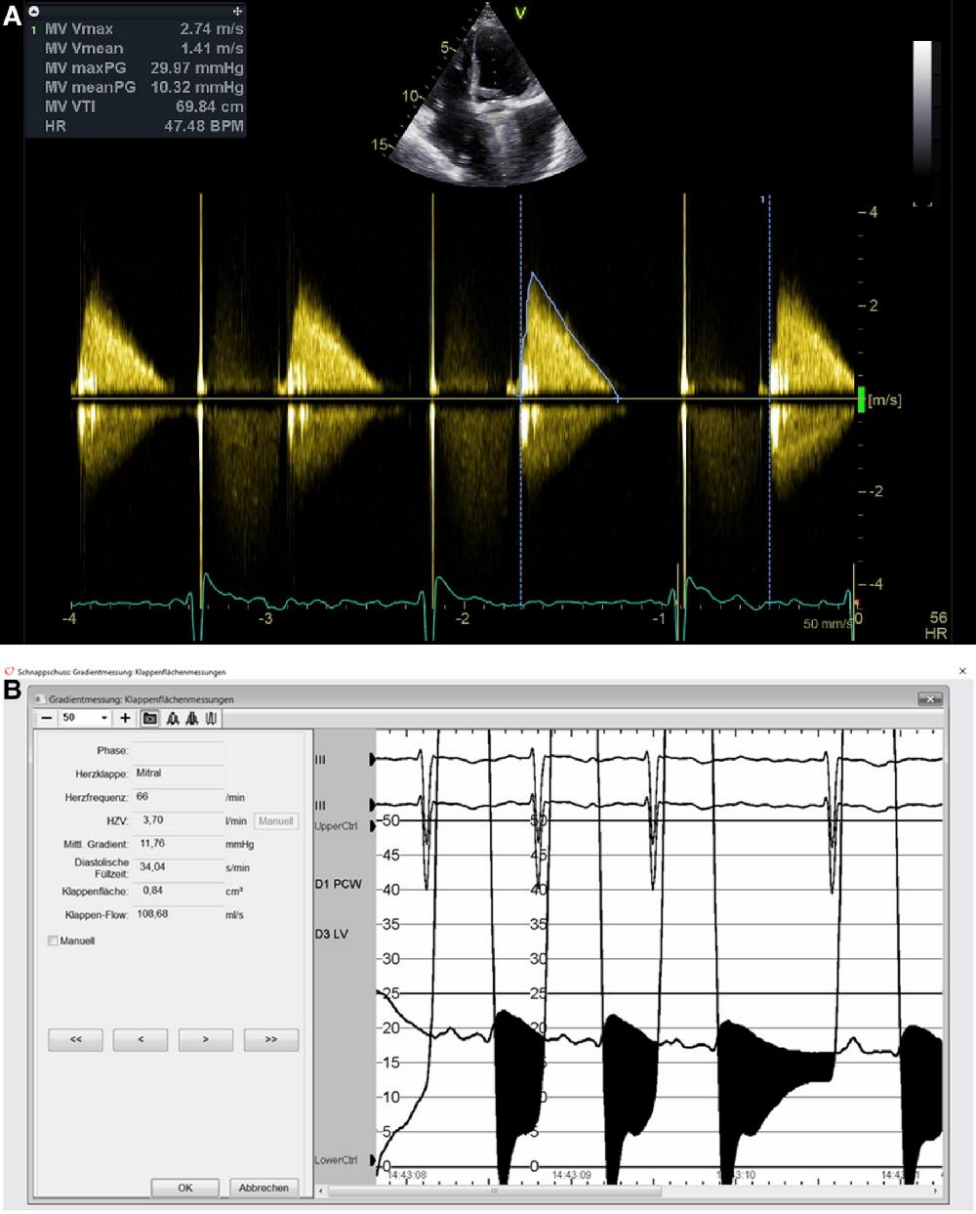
Doppler gradient of 10.32 mmHG above mitral prothesis (*A*); confirmed in invasive parallel pressure measurement (*B*).
